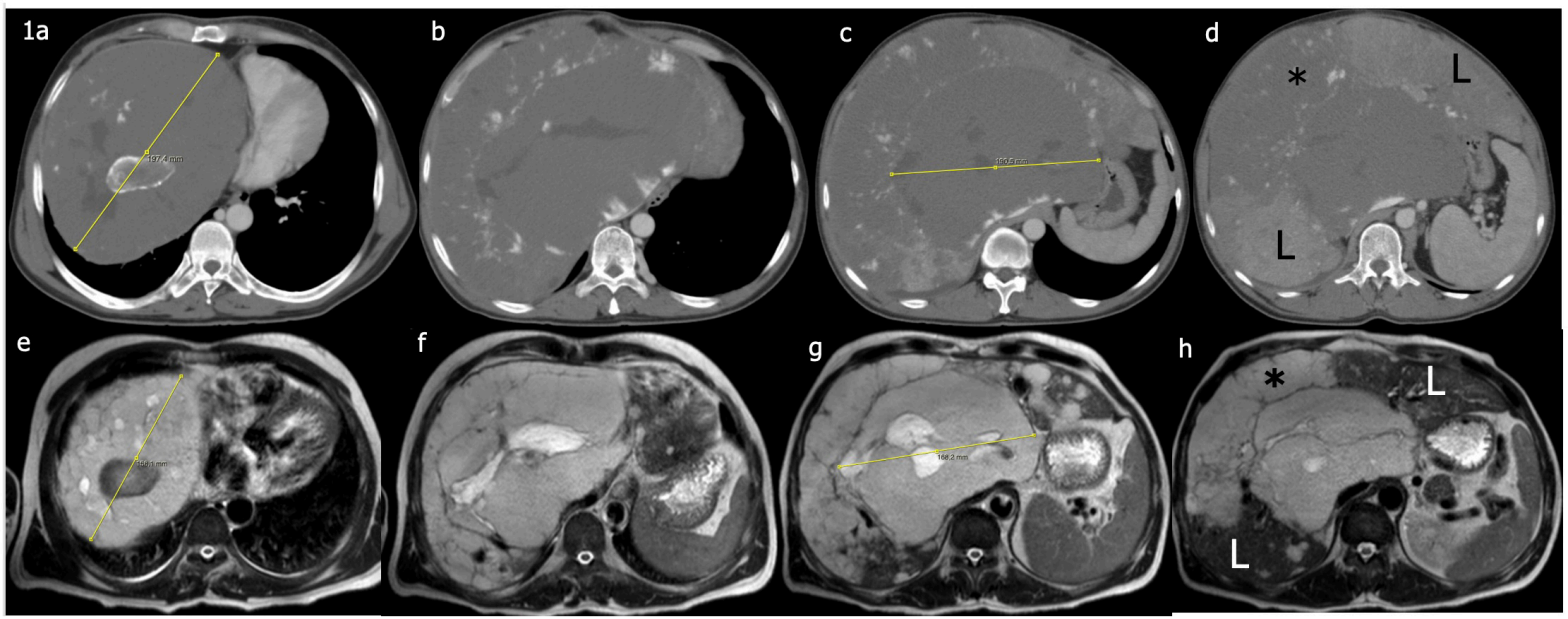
(a–d) Giant hemangioma arising from the caudate lobe, compressing the heart and segregating the right and left lobe parenchyma (L); hemangiomatous changes were evident in the adjacent areas (*). (e–h) MRI taken 4 months after TACE. Marked regression is evident. The asymptomatic patient refused further radiologic investigations.
